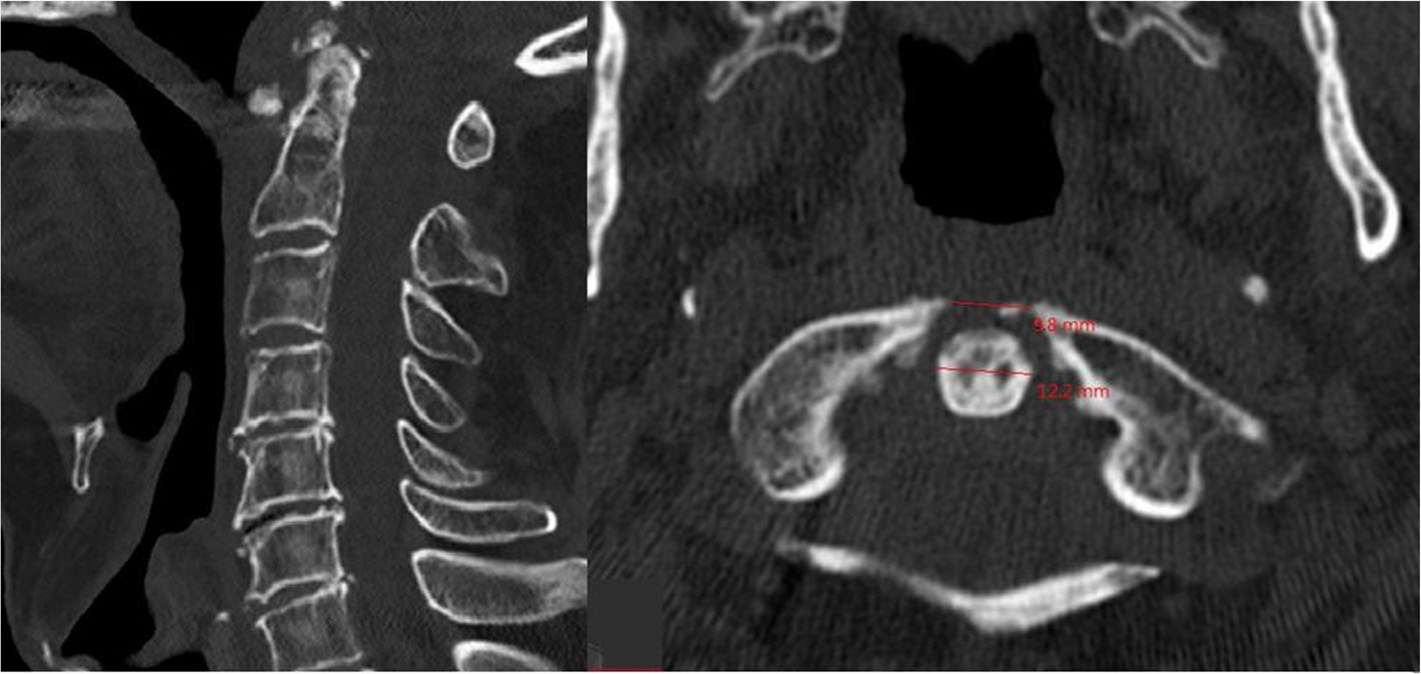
Postoperative mid-sagittal and axial CT slices showing a normalization of the C1-C2 alignment and ADI
